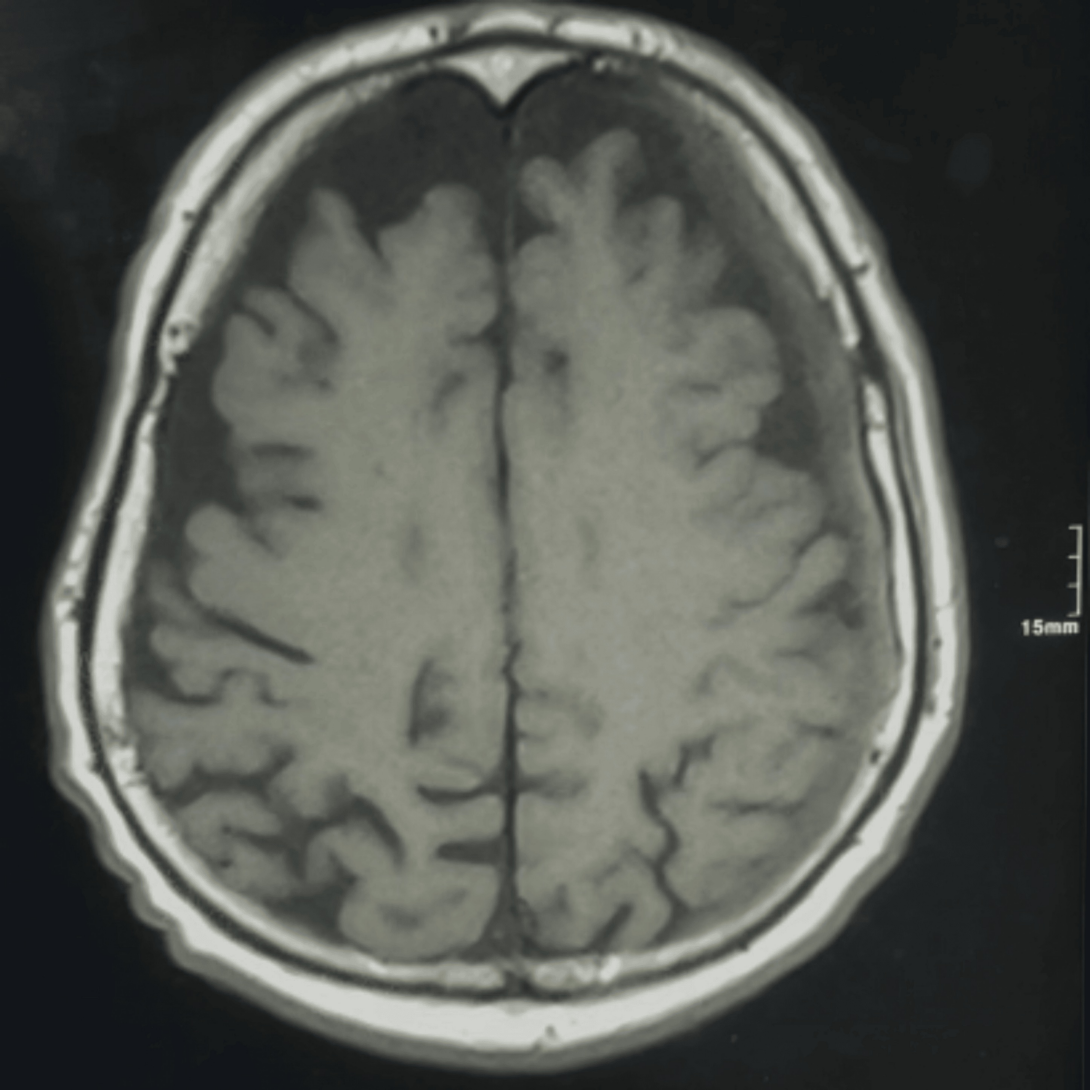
Preoperative cerebral MRI Left fronto-parietal extra-axial subdural collection suggestive of chronic subdural hematoma. MRI: magnetic resonance imaging
